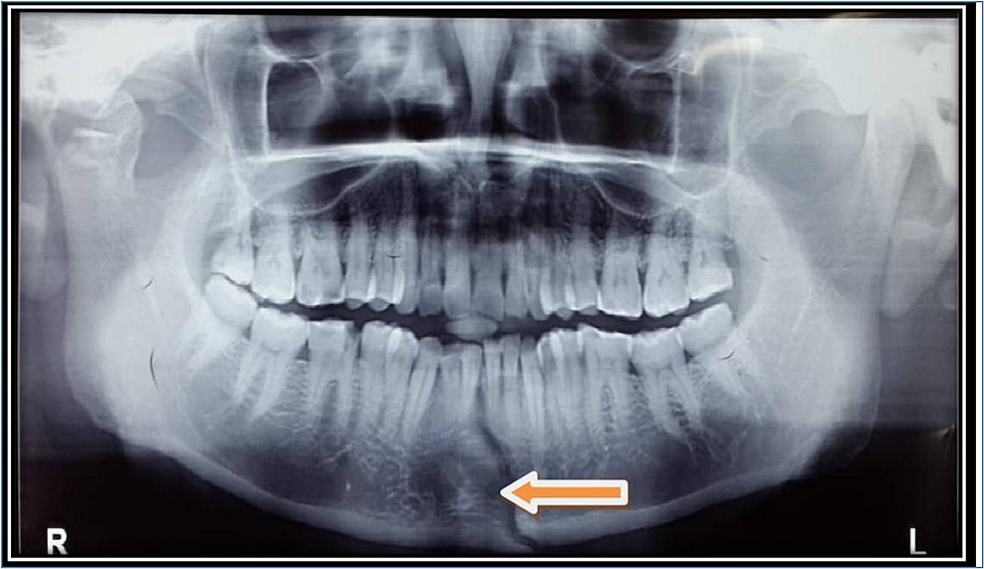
OPG showing fracture site OPG: orthopantomograph
